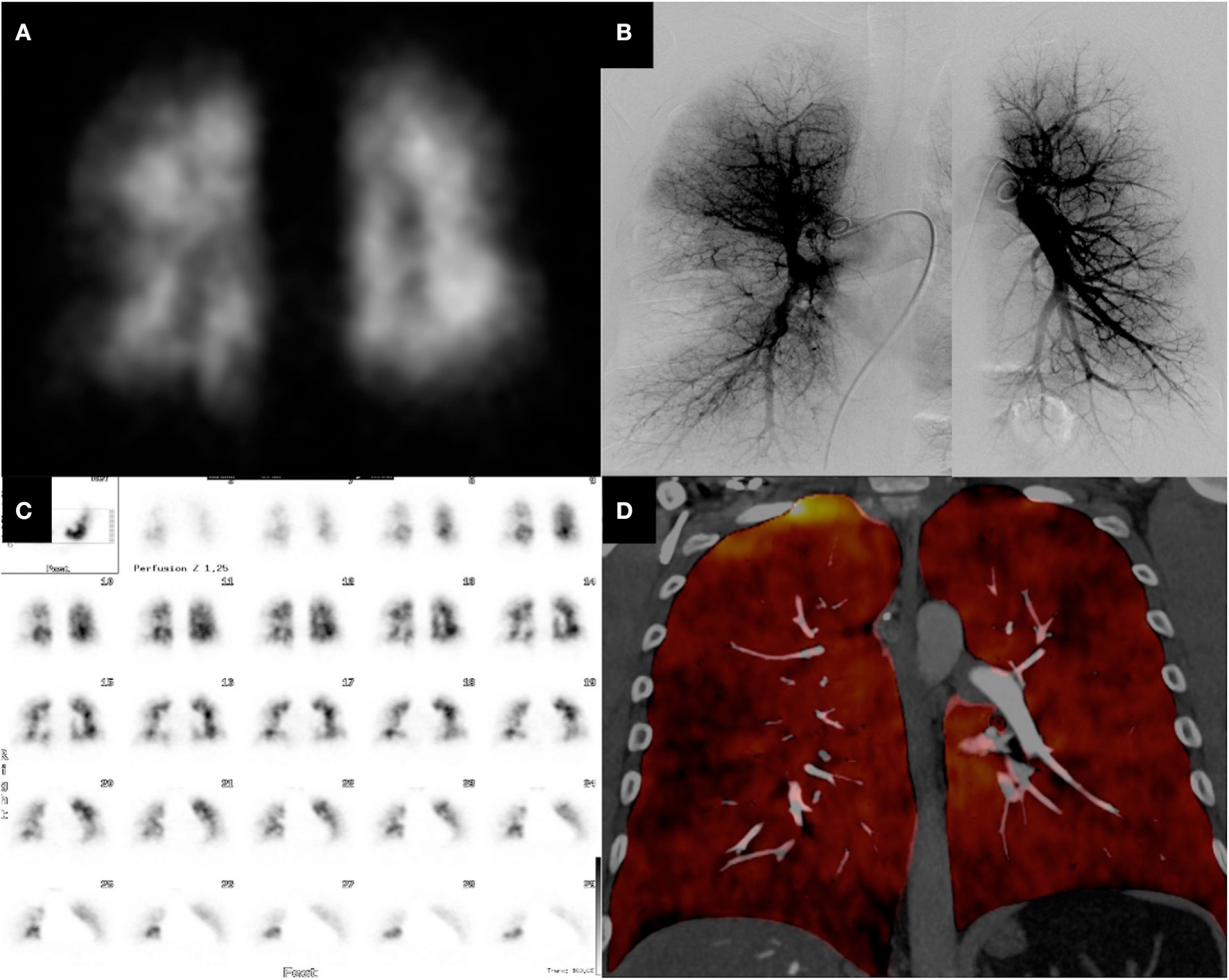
CTEPH patient with perfusion defects—DECT vs. V/Q-SPECT and PA. The figure shows the DECT (D), V/Q-SPECT (A, C), and PA (B) images of a 47-year-old male patient. All modalities show very clearly the segmental, subsegmental, and peripheral perfusion deficits.
